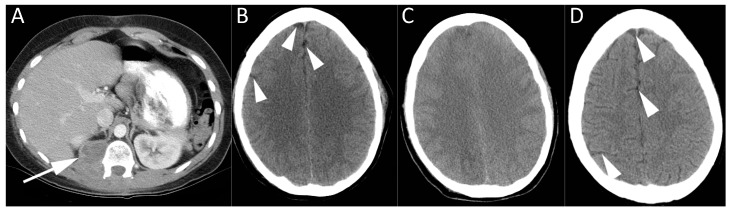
CT imaging of a 20-year-old female with paraspinal tumor seen on axial contrast enhanced CT (A). She became profoundly hypotensive during spinal surgery for resection of this malignant nerve sheath tumor. Air was noted in an infusion bag. CT brain at baseline (B) was normal, revealing normal volume of cerebrospinal fluid (CSF) around the cerebral hemispheres (arrowheads). Serial CT studies of the brain performed over the coming days revealed progressively worsening cerebral edema. CT brain at three days (C) shows effacement of CSF and diffuse cerebral swelling. She was managed with hyperbaric oxygen treatment and an intracranial pressure monitor was placed. CT brain performed 19 months later showed essentially normal appearances of the brain (D), with return of normal CSF spaces (arrowheads). The patient recovered from the air embolism, but died 25 months later from metastatic disease.
